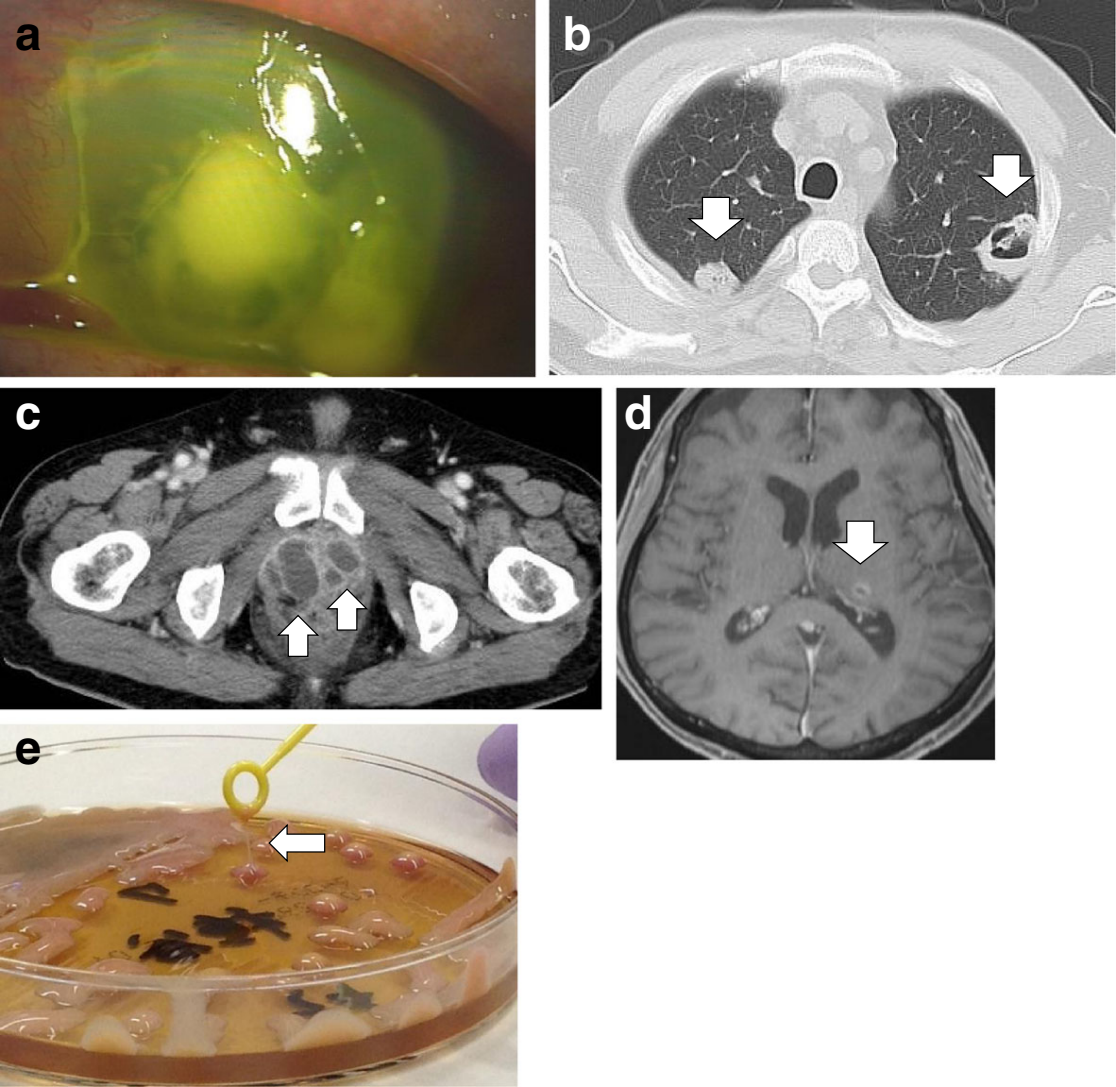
Findings from the second case. a Findings of remarkable edema and opacity in the cornea. b Contrast-enhanced whole-body computed tomography (CT) reveals lung abscesses (*white arrows*). c Contrast-enhanced whole-body CT reveals prostate abscesses (*white arrows*). d Contrast-enhanced head magnetic resonance imaging reveals a brain abscess (*white arrow*). e The second case provided colonies on the agar plate that stretched for >5 mm using the inoculation loop (*white arrows*), which indicates a positive string test result
